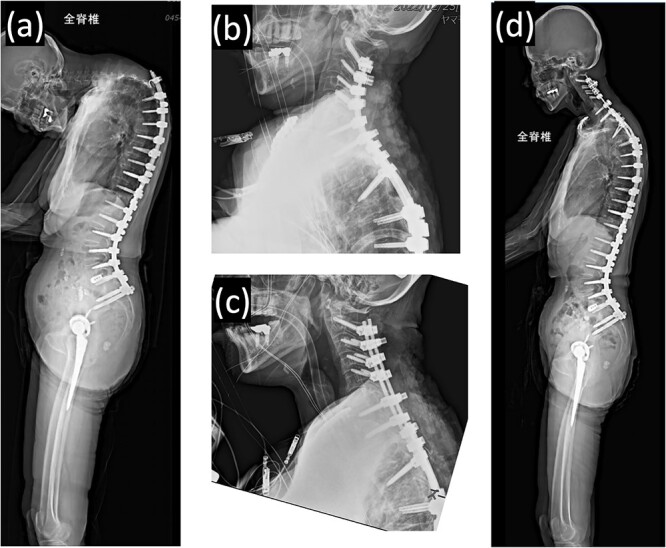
(a) Standing whole-spine radiographs 16 months after the first surgery, showing features of dropped head syndrome associated with proximal junctional failure; lateral radiographs immediately after (b) the second and (c) third surgeries; (d) standing whole-spine radiographs 16 months after the third surgery.
